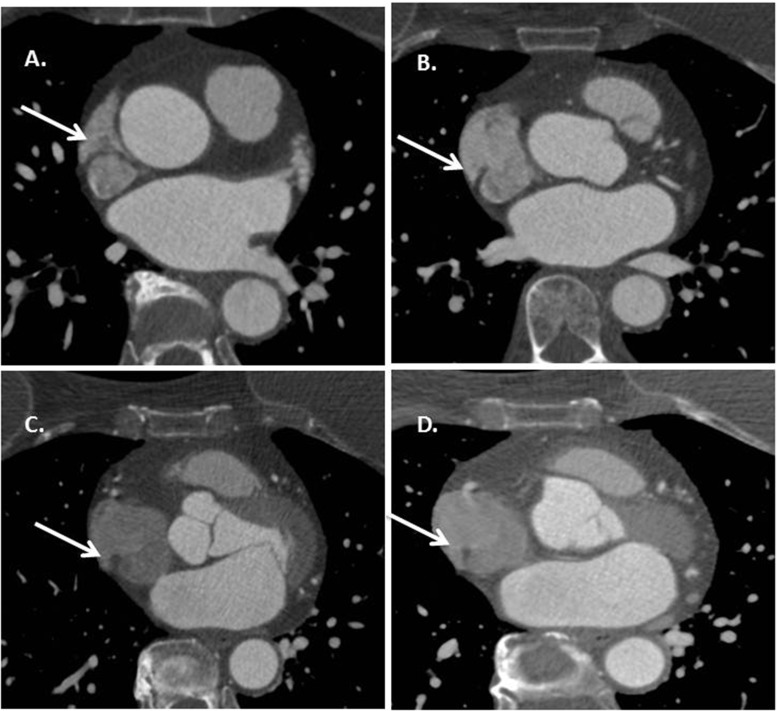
Crista terminalis. Serial axial images (a-d) of a coronary CT angiography study demonstrating the normal appearance of the crista terminalis (arrow) extending in the cranio-caudal region in the posterior wall of the right atrium
